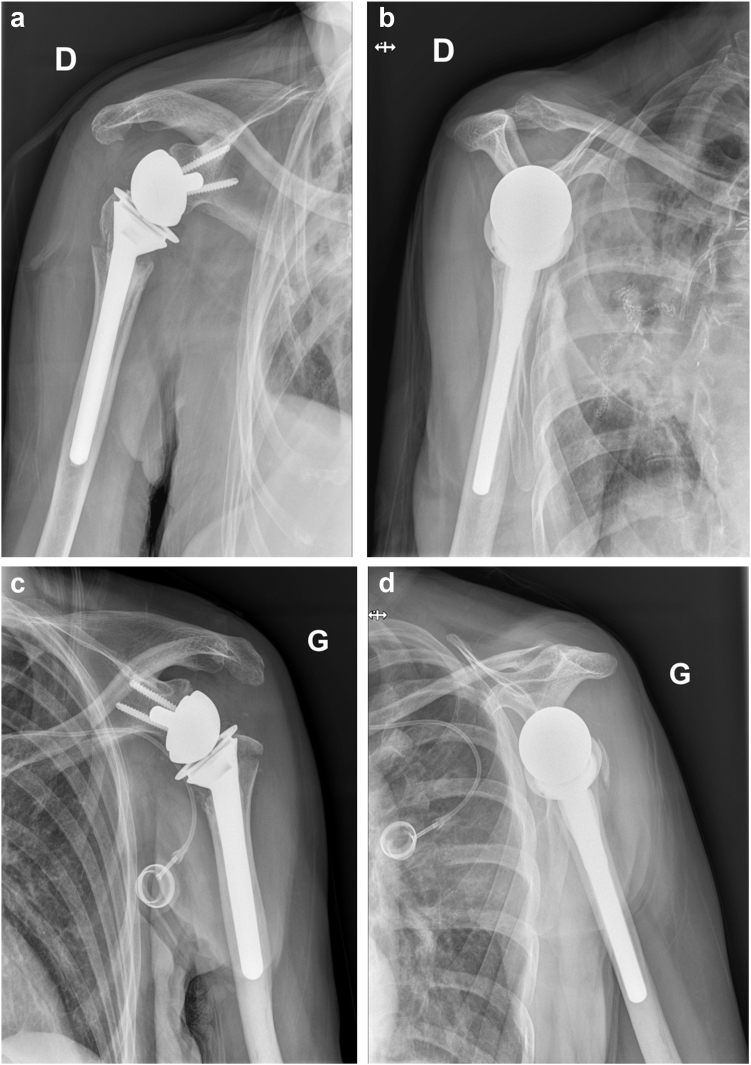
X-ray (anteroposterior, Neer views) 6 weeks postoperative of Right (a, b) shoulder and Left, (c, d) shoulder, showing correct positioning of reverse total shoulder arthroplasty on both sides.
